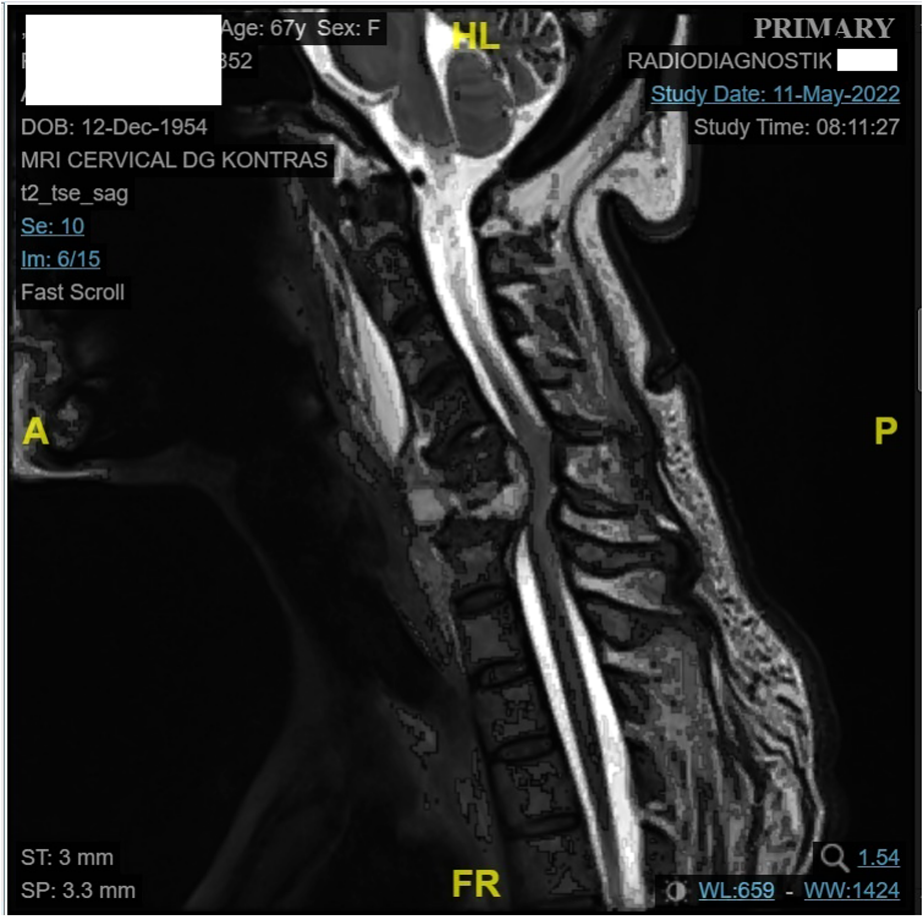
MRI of the patient. Vertebral body destruction of C5 and C6 accompanied by signs of myelopathy, suggestive paravertebral abscess measuring 1.54 x 3.50 x 1.67 cm, infiltrating m. long of neck bilateral, retrolisthesis of the C6 to C7 vertebral bodies posteriorly with a displacement of >50%. Supporting the diagnosis of SCI of our patient.
